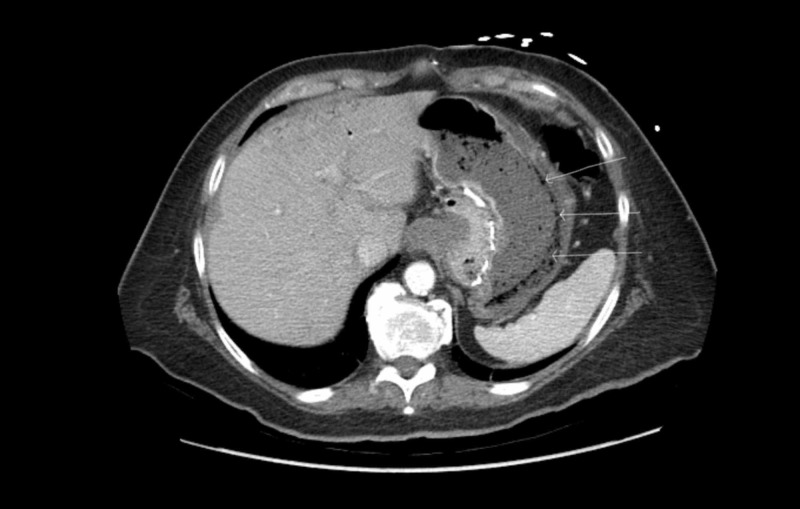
Computed tomography (CT) of the abdomen showing gastric emphysema (arrows)
